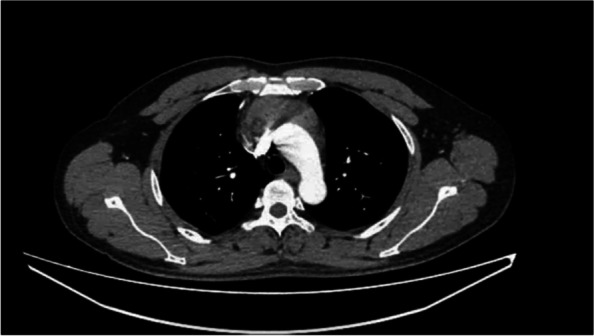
Contrast-enhanced CT scan showing partial enhancement in soft tissue, measuring 7 cm × 6 cm in size, in the anterior mediastinum
